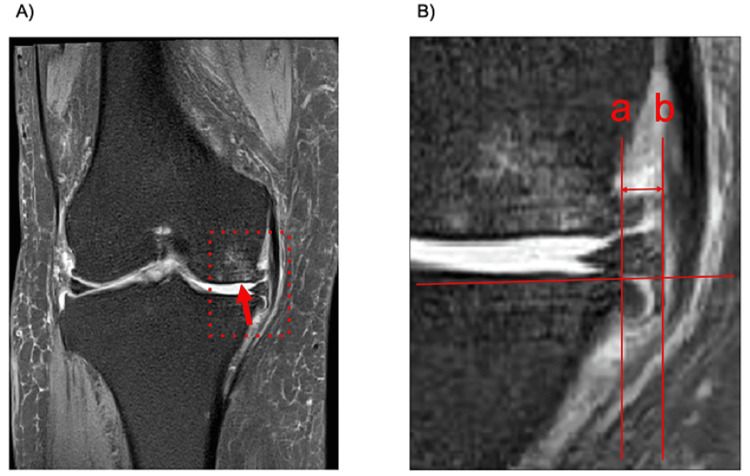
Imaging data analysis with MRI pictures. A) One of the representative images showing the bone marrow lesion in the medial femoral epicondyle (arrow). B) Enlarged image of the area enclosed by the red dotted line in the image (A). Medial meniscus extrusion is determined by measuring the distance between a line (a) perpendicular to the line connecting the medial and lateral edges of the tibial articular surface and passing through the medial edge of the medial tibial joint surface and a line (b) passing through the medial edge of the medial meniscus. MRI: magnetic resonance imaging
